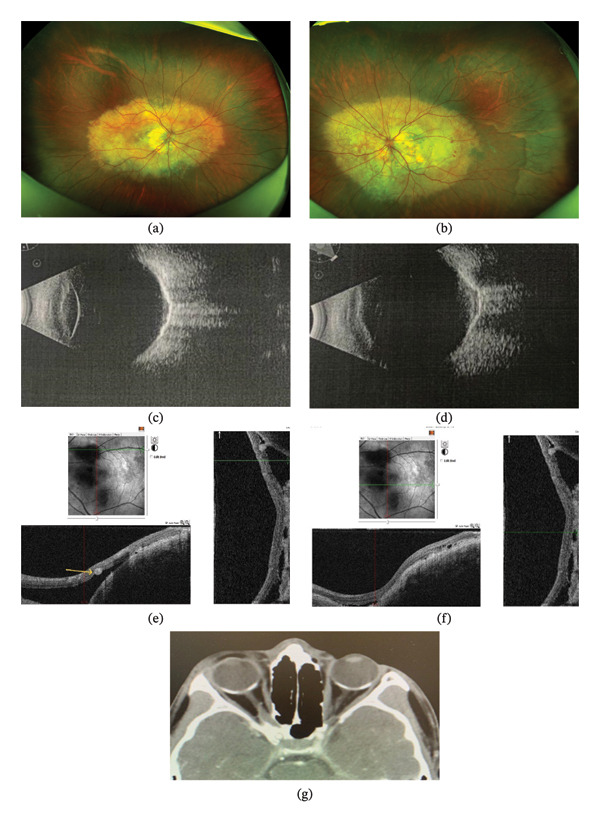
Clinical Case 2: (a, b) fundus photographs of both eyes—a peripapillary yellowish lesion with macular involvement, (c, d) B‐scan ultrasound of both eyes—increased echogenicity of the lesions is observed, OCT of the right eye, (e) the retinal profile is deformed by a prominent subretinal lesion with increased hyperreflectivity at the retinal pigment epithelium—Bruch’s membrane complex. A localized microcystic intraretinal edema is visualized over the area of prominence, (f) a hyperreflective lesion corresponding to a calcification (indicated by the yellow arrow) is observed extending from the outer plexiform layer to the ellipsoid zone of the neuroepithelium, (g) CT scan of the orbits of both eyes—CT signs of calcification in the posterior poles of both eyes.
